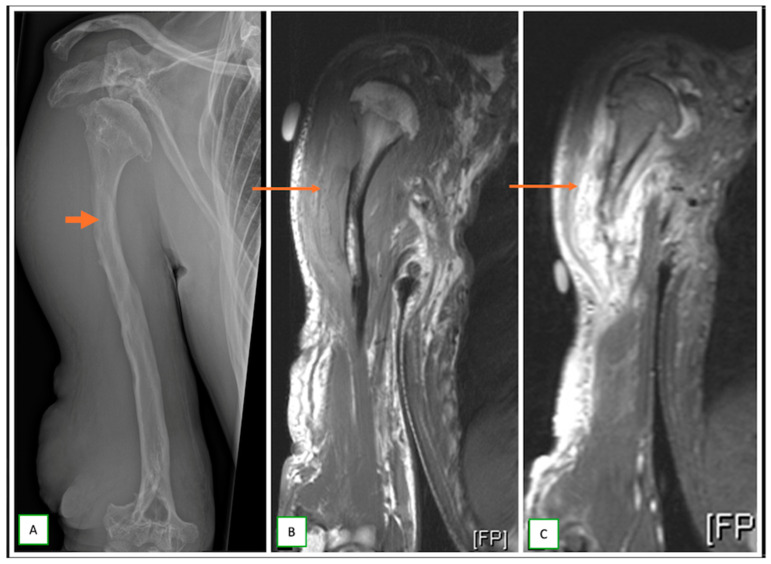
Plexiform neurofibroma in a 75-year-old man with a case of neurofibromatosis type 1 who presented with a right arm painful lump. The frontal radiograph of the right humerus (A) shows a congenital dysplasia/remodeling with a bowed gracile right humerus (Thick arrows). Coronal T1 (B) and coronal fat-saturated T2 (C) images show a plexiform neurofibroma (Thin arrows) which appears as a diffusely long segment enlargement of multiple arm nerves akin to a “bag of worms”.
